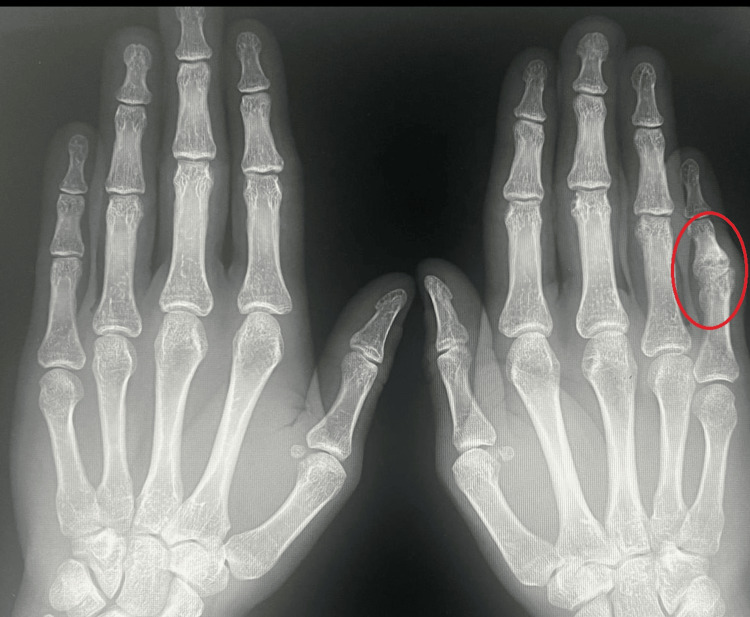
X-ray of both hands before surgery in AP projection Red circle indicates the proximal interphalangeal joint. AP: anteroposterior.
